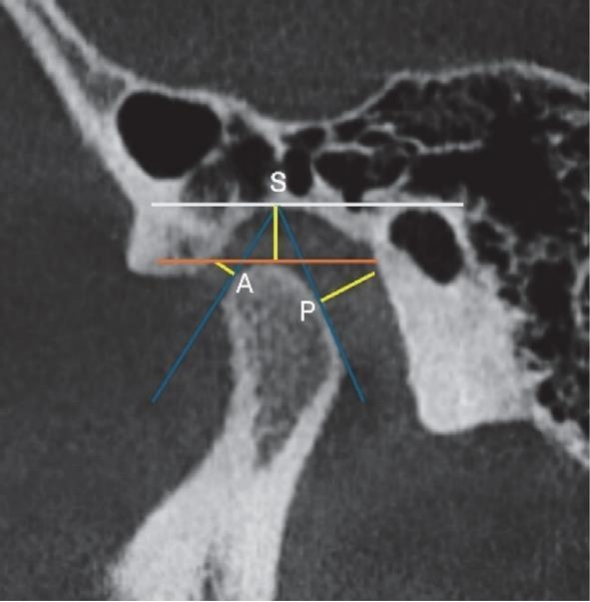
Sagittal section of TMJ in CBCT showing reference points for measurement of AJS, SJS, and PJS.
